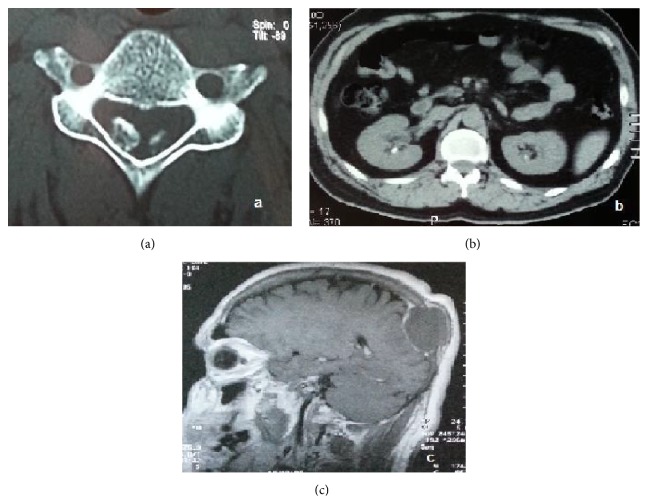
A 45-year-old man with parathyroid carcinoma: (a) ossification of the cervical ligamentum flavum in C2-C3 and C4-C5 without signs of myelopathy. (b) Nephrolithiasis in both kidneys and (c) left parietal bone tumor (5.5 × 3.2 × 4.4 cm) from Magnetic Resonance Imaging scan.
